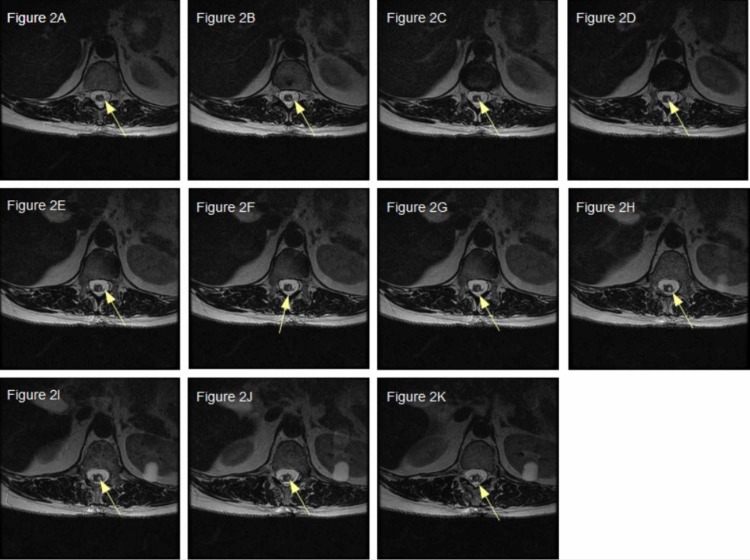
Iatrogenically-induced syrinx (A-K) Consecutive, rostral to caudal, axial T2-weighted MRI images with yellow arrows directed at T2 hyperintensity representing the syrinx between T11 and T12.
